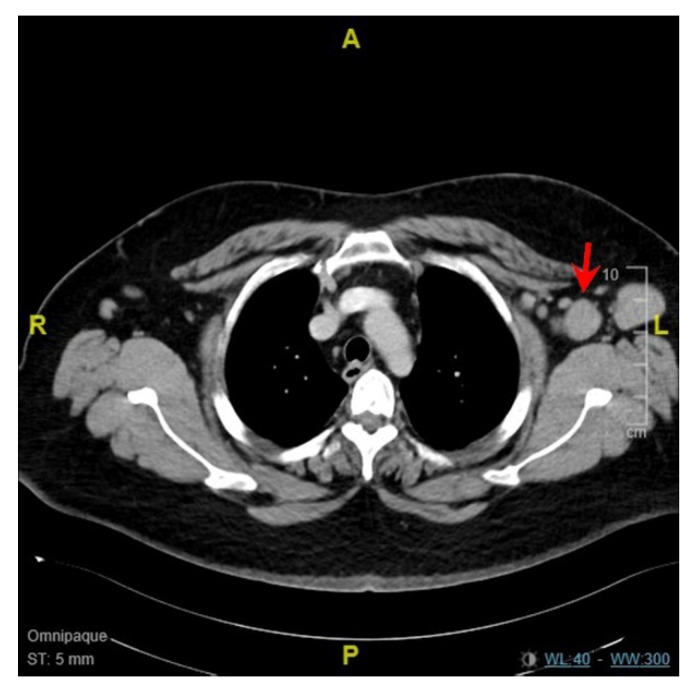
Computed tomography scan of patient B demonstrating enlarged left axillary lymph nodes (red arrow).
